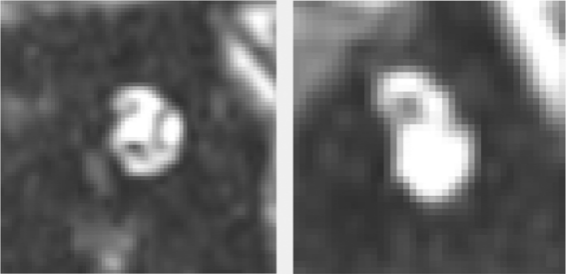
MRI, T2 image of a transversal section through the internal auditory meatus. Aplasia of the hearing nerve on the right side (right image) and normal findings on the left with acoustic nerve (left below), facial nerve (left above), and vestibular nerve (right)
